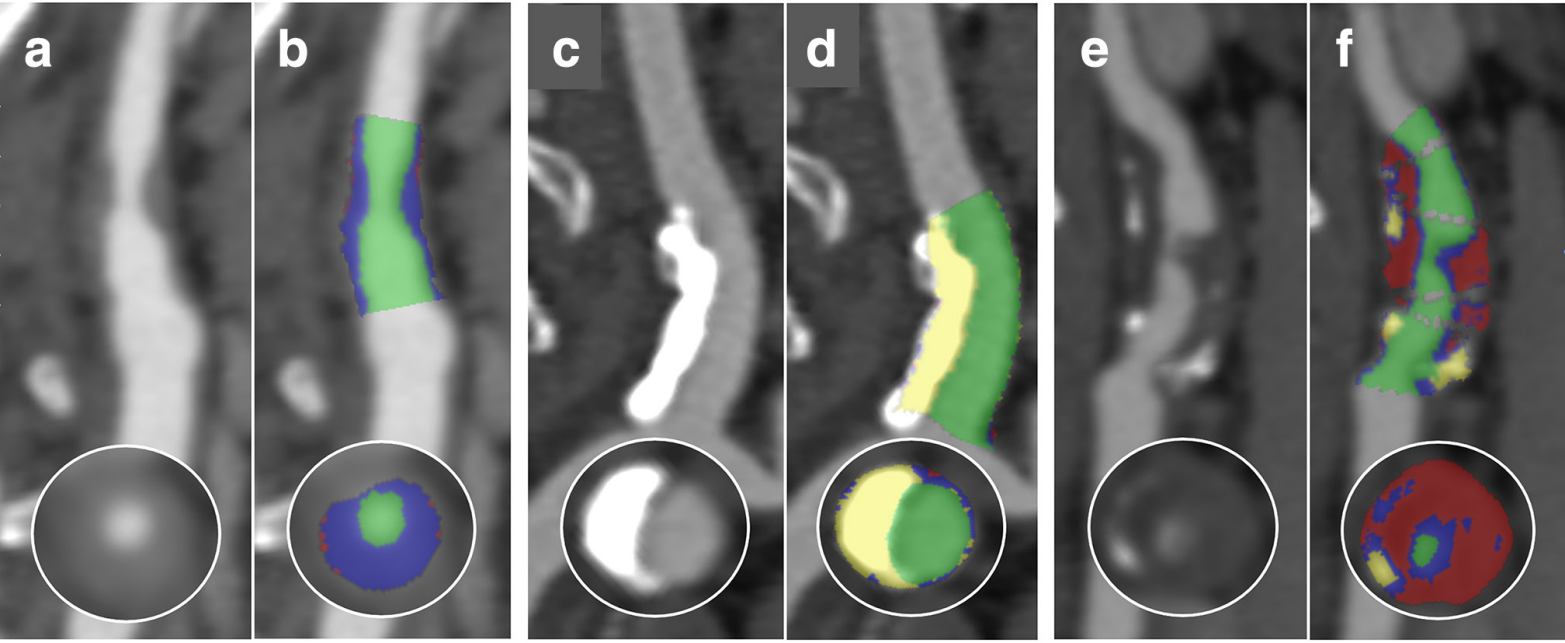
Conventional multiplanar reformat and CT Plaque Map analysis of (a) and (b) predominantly fibrous plaque, (c) and (d) calcified plaque and (E) and (F) necrotic core. With fibrous plaque colored blue, calcified plaque white, necrotic core red and lumen green.
